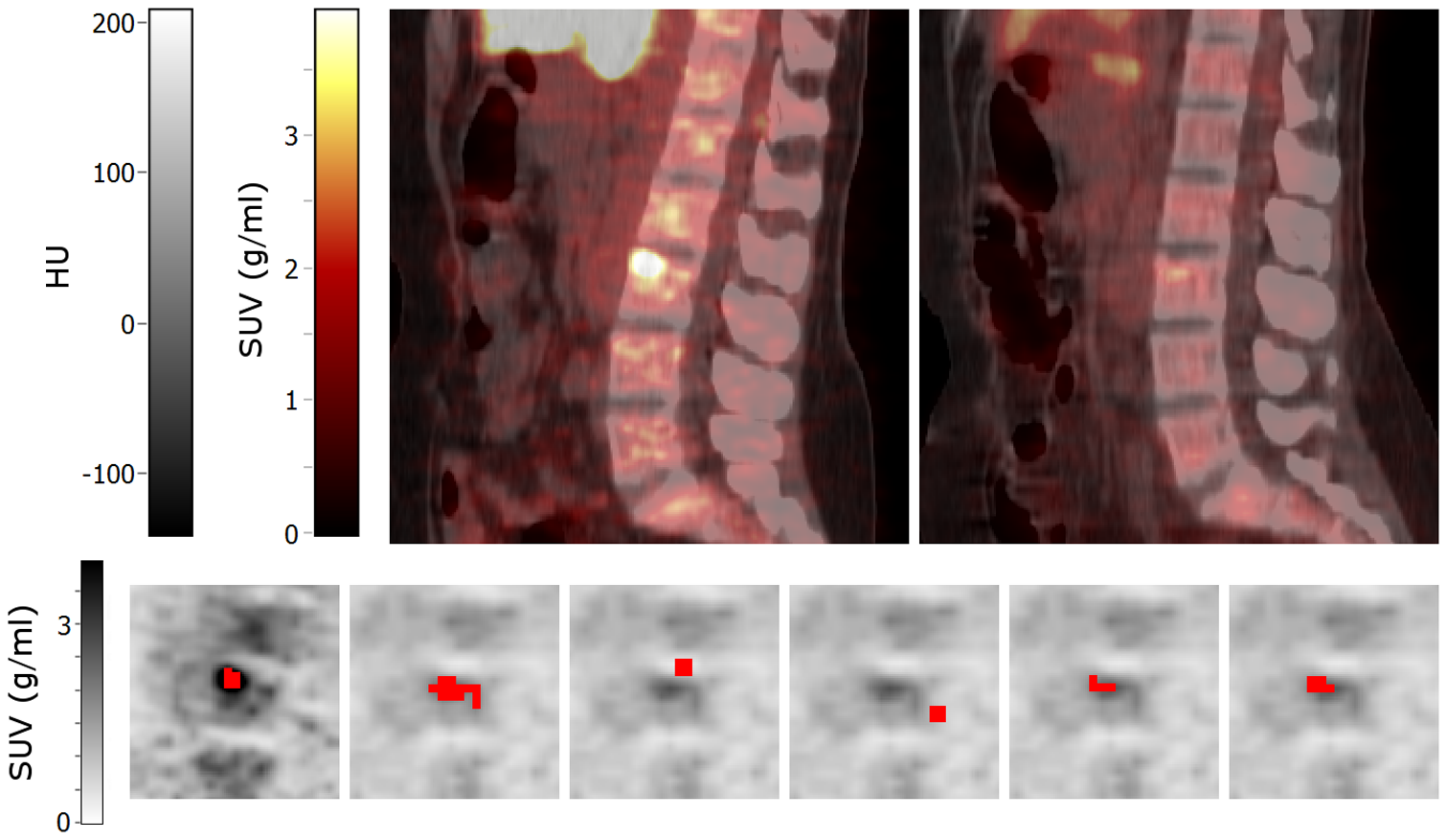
Sagittal images of a patient with a bone metastasis. Top row: baseline (left) and early response (right) PET/CT images. Bottom row: volumes of interest (shown in red) projected onto the baseline (first image) and early response scans (other images) that were obtained using (from left to right): A50 defined on baseline scan, A50 defined on early response scan, and global rigid PET, local rigid CT, non-rigid PET and non-rigid CTPET image registration. All images are shown using the same colour scales. Abbreviations: SUV, standardized uptake values; HU, hounsfield units; A50, 3 dimensional (semi-)automatic isocontour method at 50% of the maximum pixel value that corrects for local background.
